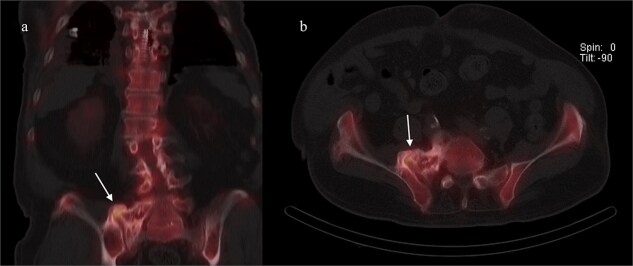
Coronal (left) and axial (right) views of pelvic CT-SPECT highlighted increased uptake along the right SI joint, suggestive of inflammation (arrows).
